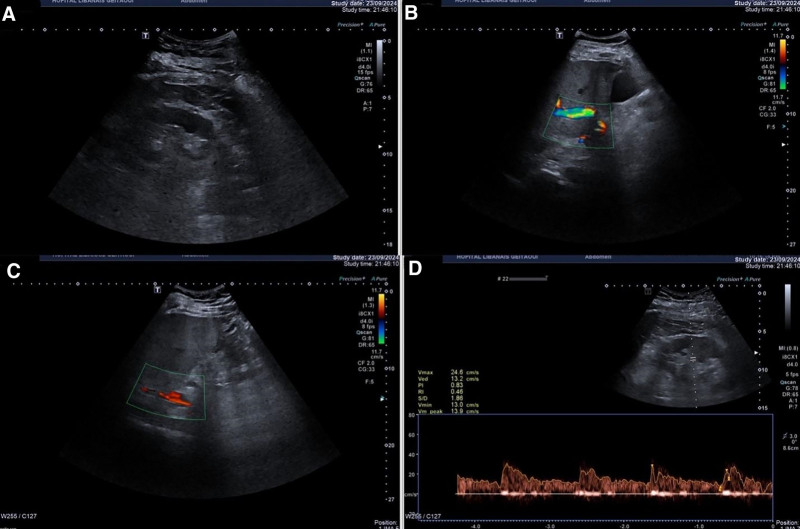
(A–D) Doppler ultrasound of the abdomen and pelvis with arterial and venous spectral waveforms at the level of the kidneys showing no evidence of renal vein thrombosis.
